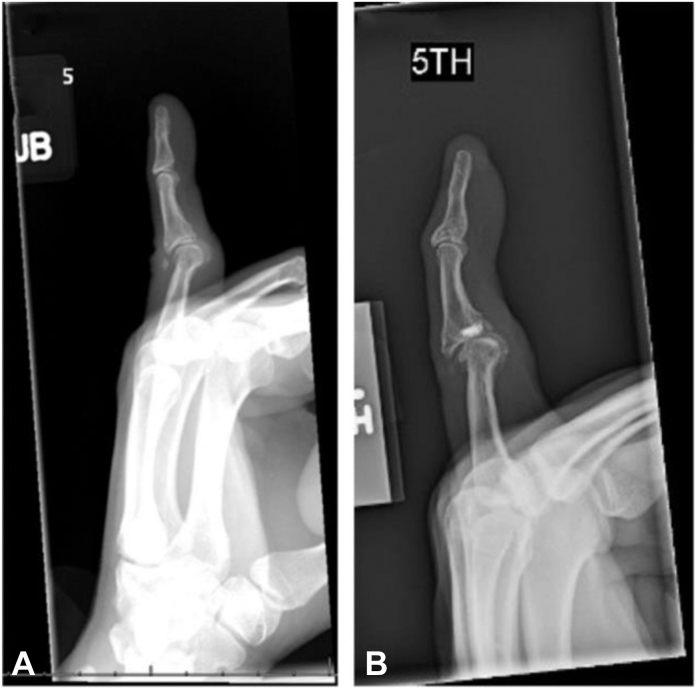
A Preoperative radiograph of a 21-year-old patient with a chronic VP injury. B A 2.5-year postoperative radiograph of a repaired VP 30 years after injury.
